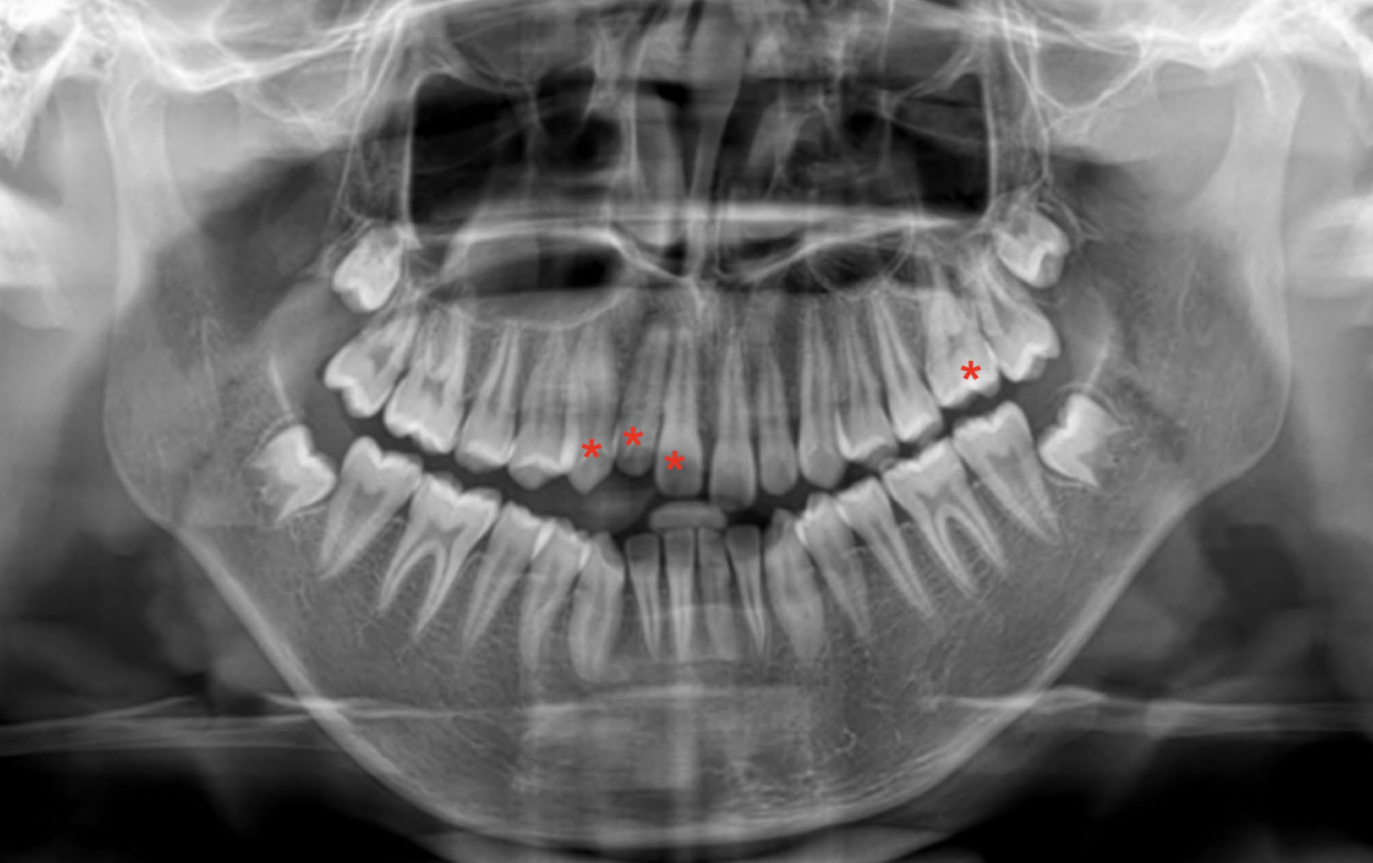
Pretreatment panoramic radiograph
